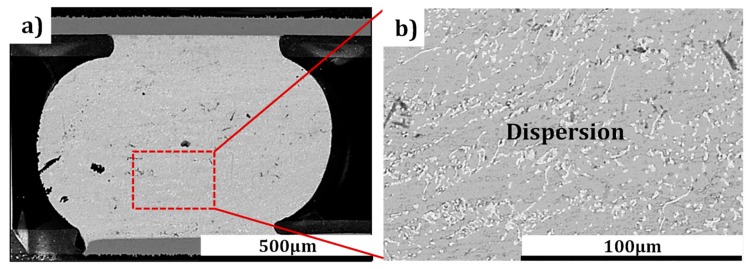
(a) and (b) are SEM images of the SnPb (Pb content is 22.46%) stored at CT (−196 °C) for 10 days without current stressing.
